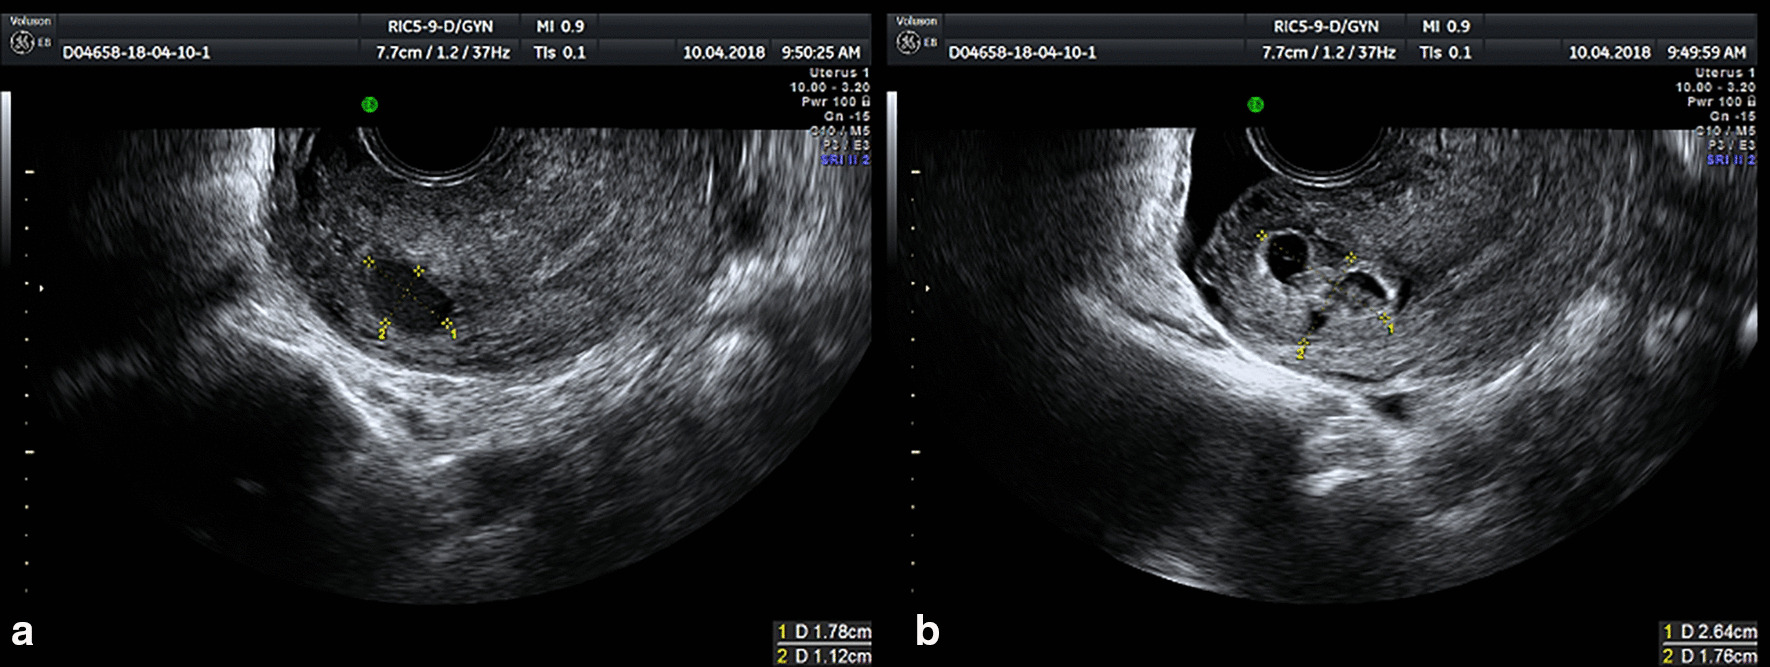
Transvaginal ultrasound image of case 1. A chaotic echo with a range of 2.64 * 1.76 cm in a clear boundary, and a liquid dark area with a range of 1.78 * 1.12 cm were found in right angular of the uterus. Gestation tissue is indicated by measurement of the lesion which looks like yellow dots
